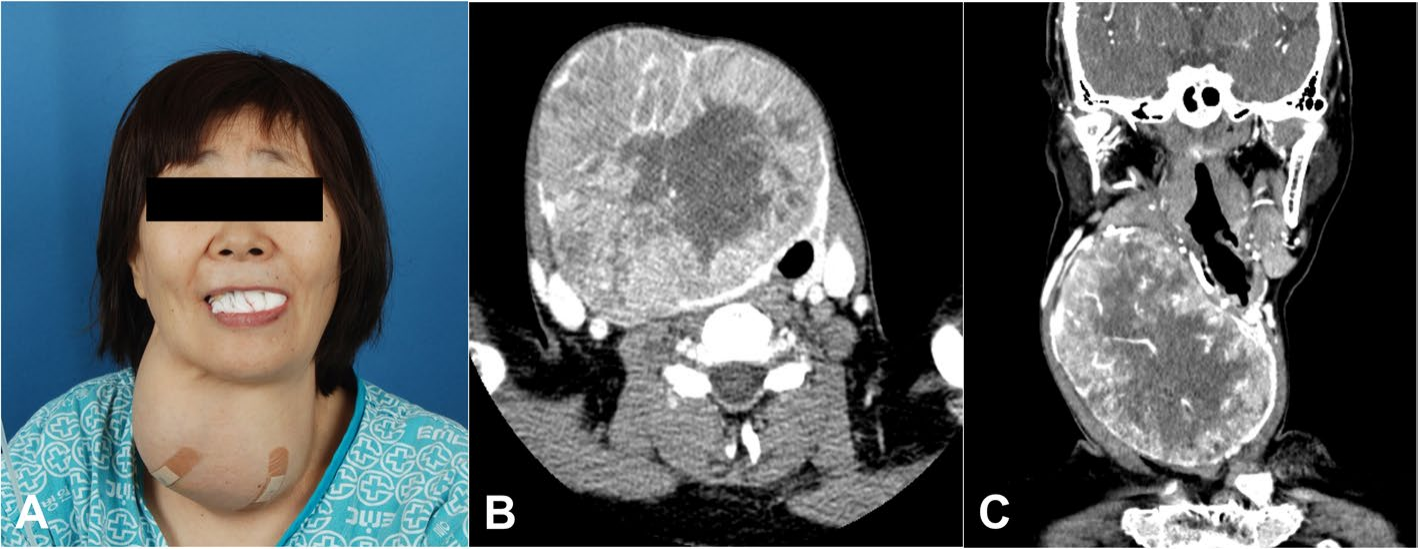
A Physical findings. An approximately 10 × 12 cm size fixed and non-tender mass is seen on anterior neck. B, C Preoperative thyroid CT. It shows a 10.9 × 9.2 cm size lobular shaped mass on the right thyroid gland, and a leftward deviation of the trachea owing to internal calcification and mass
